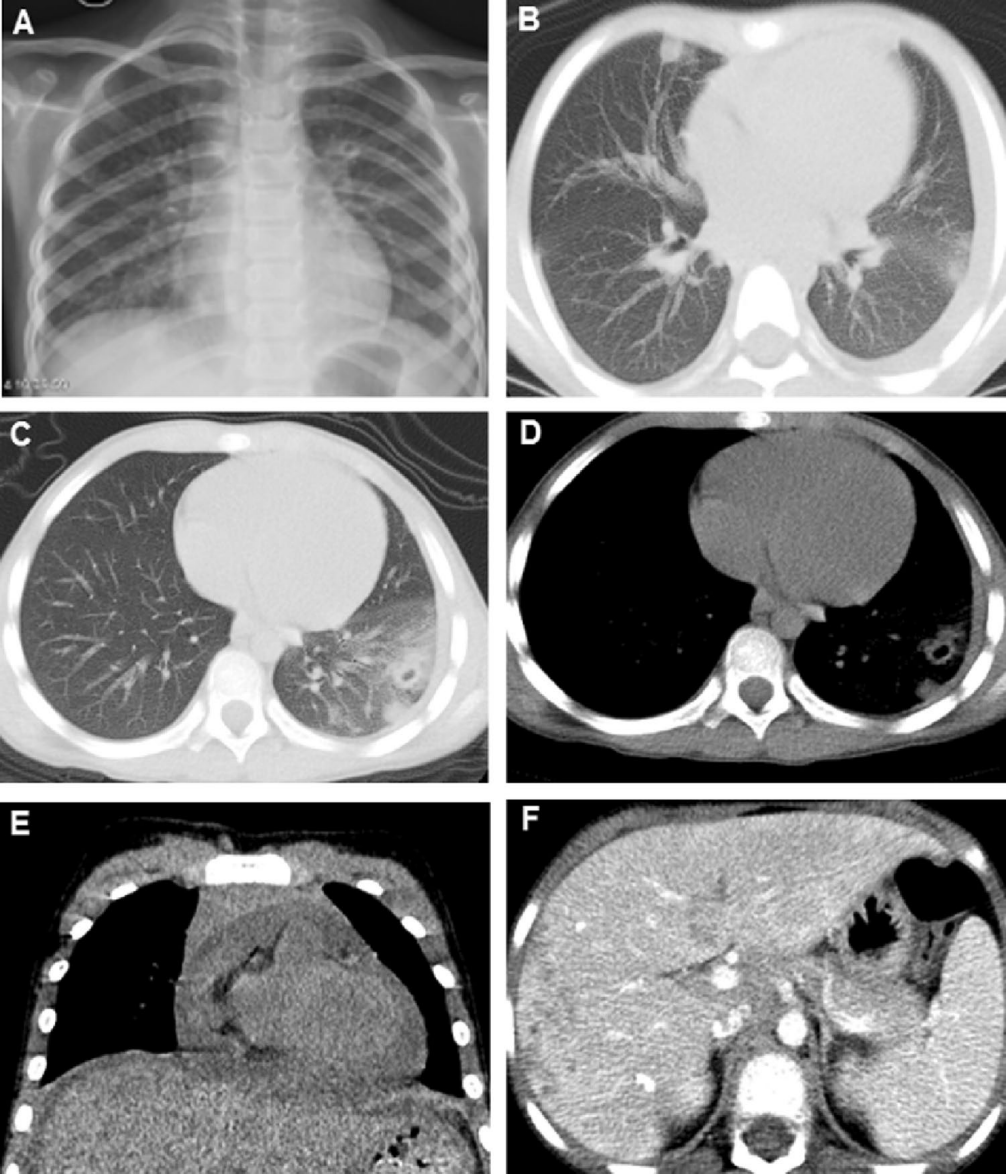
A 7-year-old girl presented with abdominal pain and subcutaneous nodules. (A) Chest X-ray showed pulmonary exudation in the right lower lung lobe; (B–E) Chest CT showed the alveolar opacification and ill-defined nodules in bilateral lungs, pleural effusion in both thoracic cavities; (F) Abdominal CT showed the multiple lesions with low density or calcifications in the liver.
